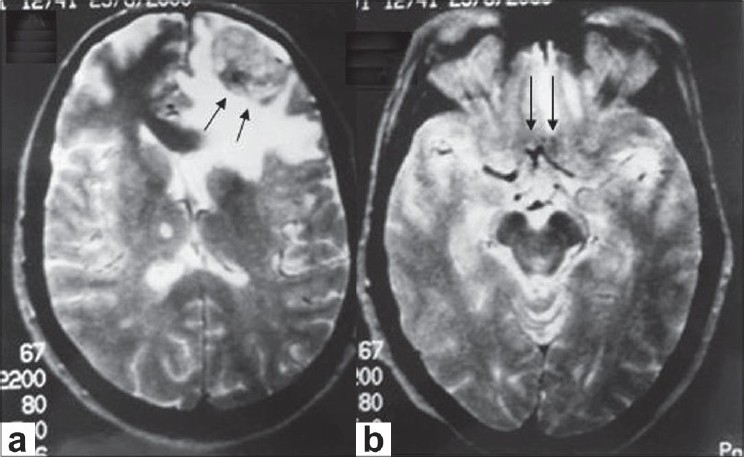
Magnetic resonance imaging brain axial section T2-weighted images showing (a) a rounded mass (arrow) in the polar region of the left frontal lobe; (b) herniation of the left gyrus rectus (arrow) resulting in obliteration of the suprasellar cistern
